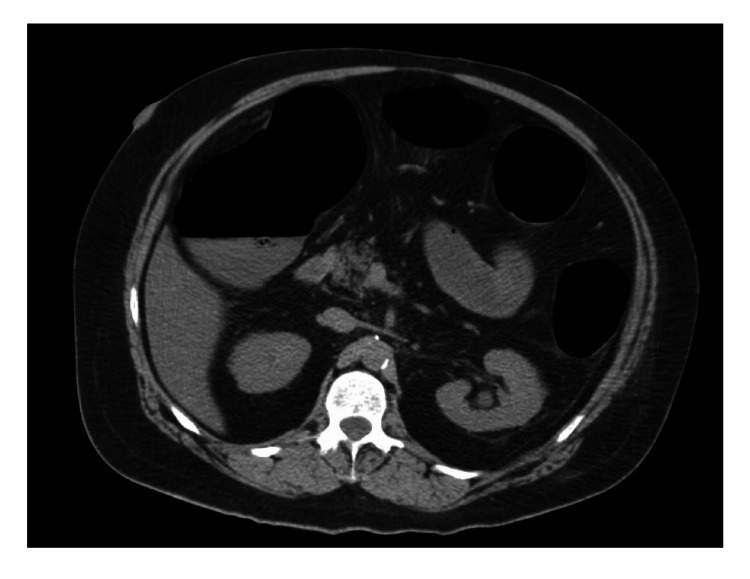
CT abdomen prior to cecostomy showing significantly distended colon suggestive of large bowel obstruction.
